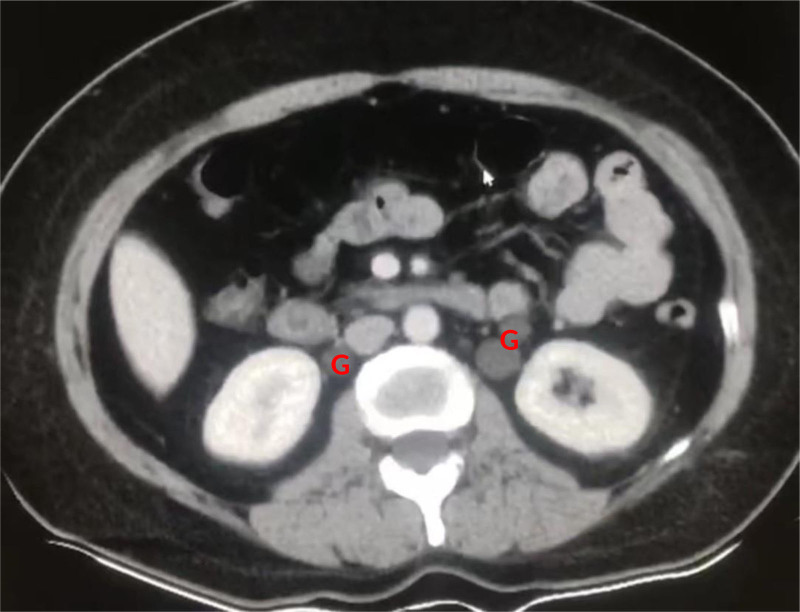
Multiple low-density masses are visible anterior to both kidneys at the level of the renal plane in the retroperitoneum (G). These masses exhibit similar CT density values to those observed in the orbits and chest lesions, and there are no enhancement changes noted after contrast administration. CT = computed tomography.
